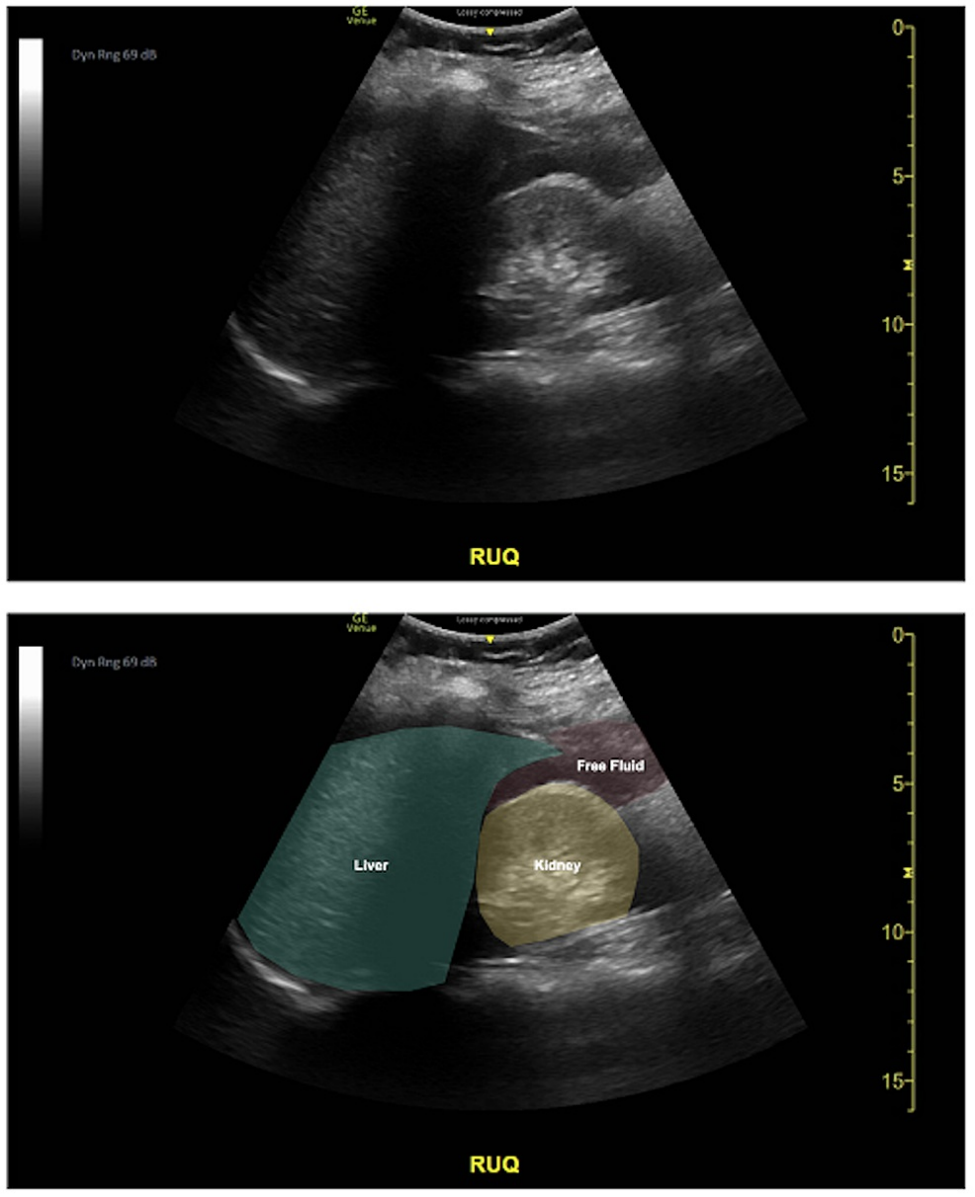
Right upper quadrant view seen on bedside ultrasonography. Transducer was placed at the mix-axillary line at approximately the 10th rib with the probe indicator towards the patient's head visualizing the diaphragm, liver, kidney and a potential space known as “Morrison’s Pouch.” This image demonstrates fluid, visualized as an anechoic (black) area inside of Morrison’s Pouch. The lower image is a color-coded version of the original image for visual interpretation.
